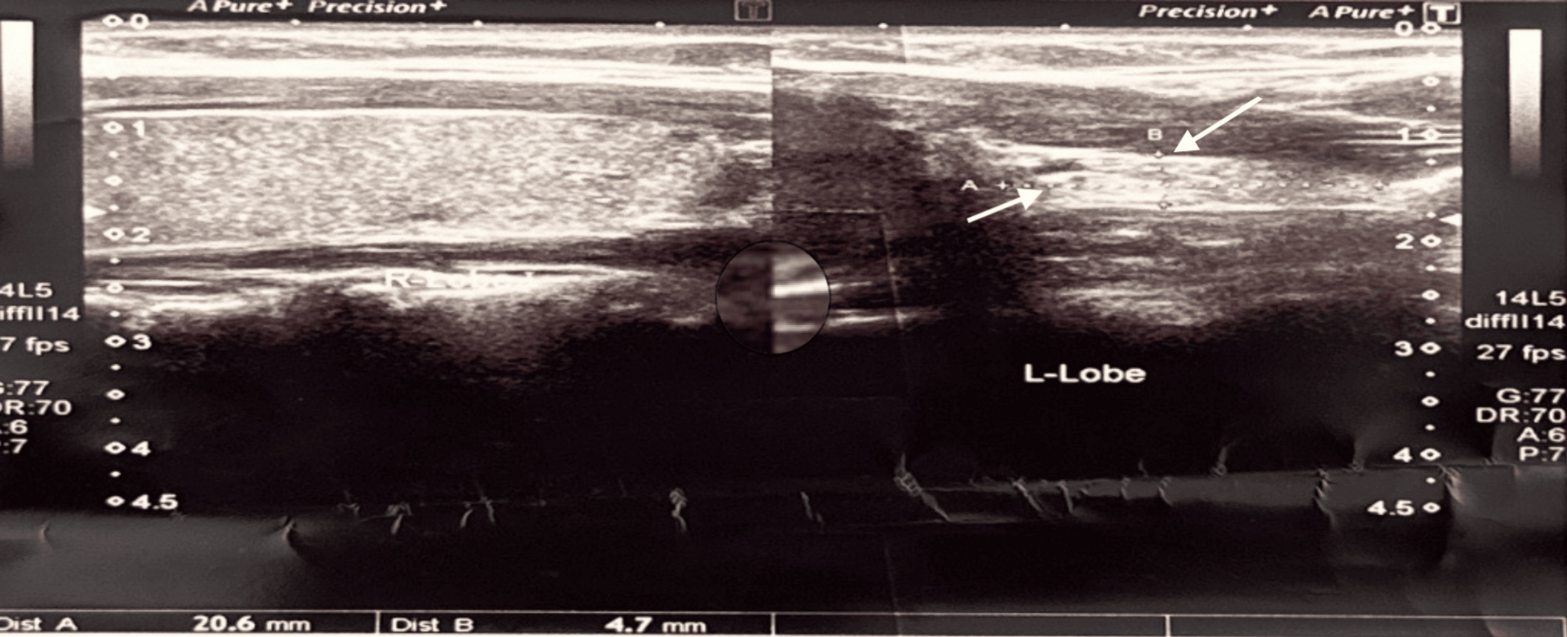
Thyroid ultrasound of a 22-year-old female showing the size of the left lobe of the thyroid, measuring 2.0 × 0.47 cm.
